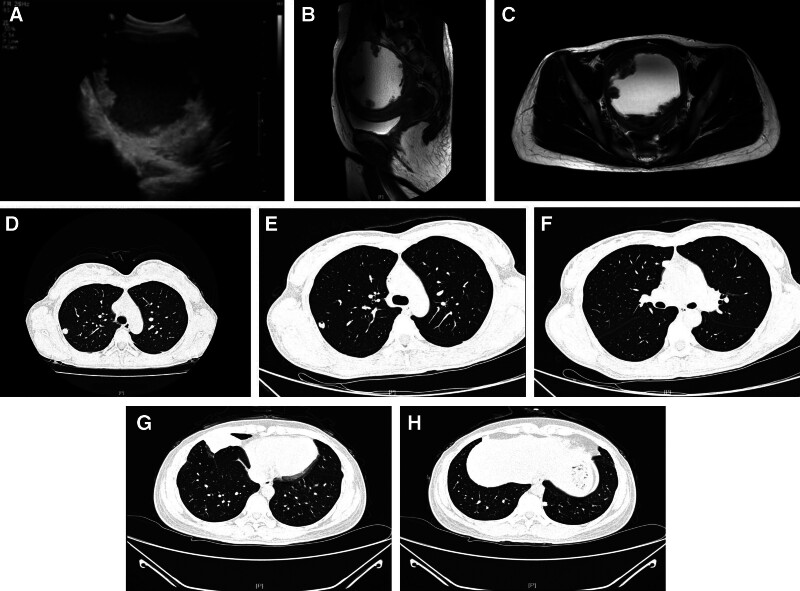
Imaging findings: (A) Ultrasound scan showed a lesion measuring 10.5*10.2 cm with blood flow; (B and C) On T2-weighted images of MRI revealed a 10.4*11.0*10.8 cm mass in the uterine posterior wall, and multiple irregular nodules were observed at the edges and protruded into the lesion; (D) The HRCT scan of the chest on July 23, 2022, showed multiple nodular hyperdensities in both lungs. The largest one about 12 mm*10 mm in size located in the right upper lobe; (E and F) The scan of preoperative HRCT showed multiple nodules in both lungs. A 11 mm nodule in the right upper lobe exhibited an empty bubble within it. (G) The HRCT scan of chest showed multiple soft-tissue densities of both lungs under the pleura which the largest one about 55*34 mm in size located in the subpleural region of right middle lobe. (H) Multiple nodular hyperdensities were revealed in both lungs. The largest one measuring approximately 8mm in diameter located in the left lower lobe. HRCT = high-resolution computed tomography, MRI = magnetic resonance imaging.
